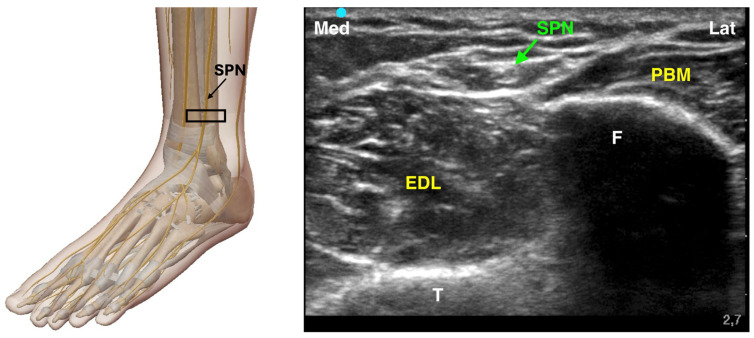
Ultrasound-guided Superficial Peroneal Nerve Block. SPN: Superficial Peroneal Nerve; F: Fibula; T: Tibia; EDL: Extensor Digitorum Longus Muscle; PBM: Peroneus Brevis Muscle; The black rectangle indicates the placement of the ultrasound probe on the anatomical area.
